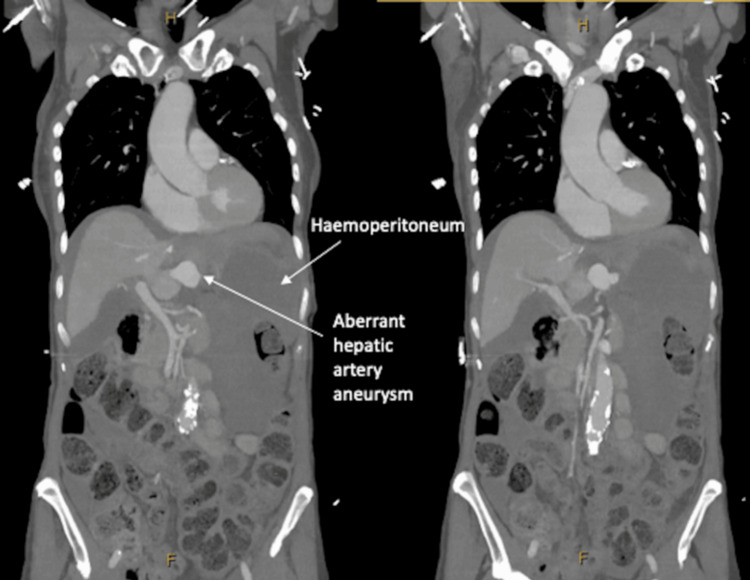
Coronal phase of CT aortogram demonstrating haemoperitoneum and aberrant left hepatic artery aneurysm.
